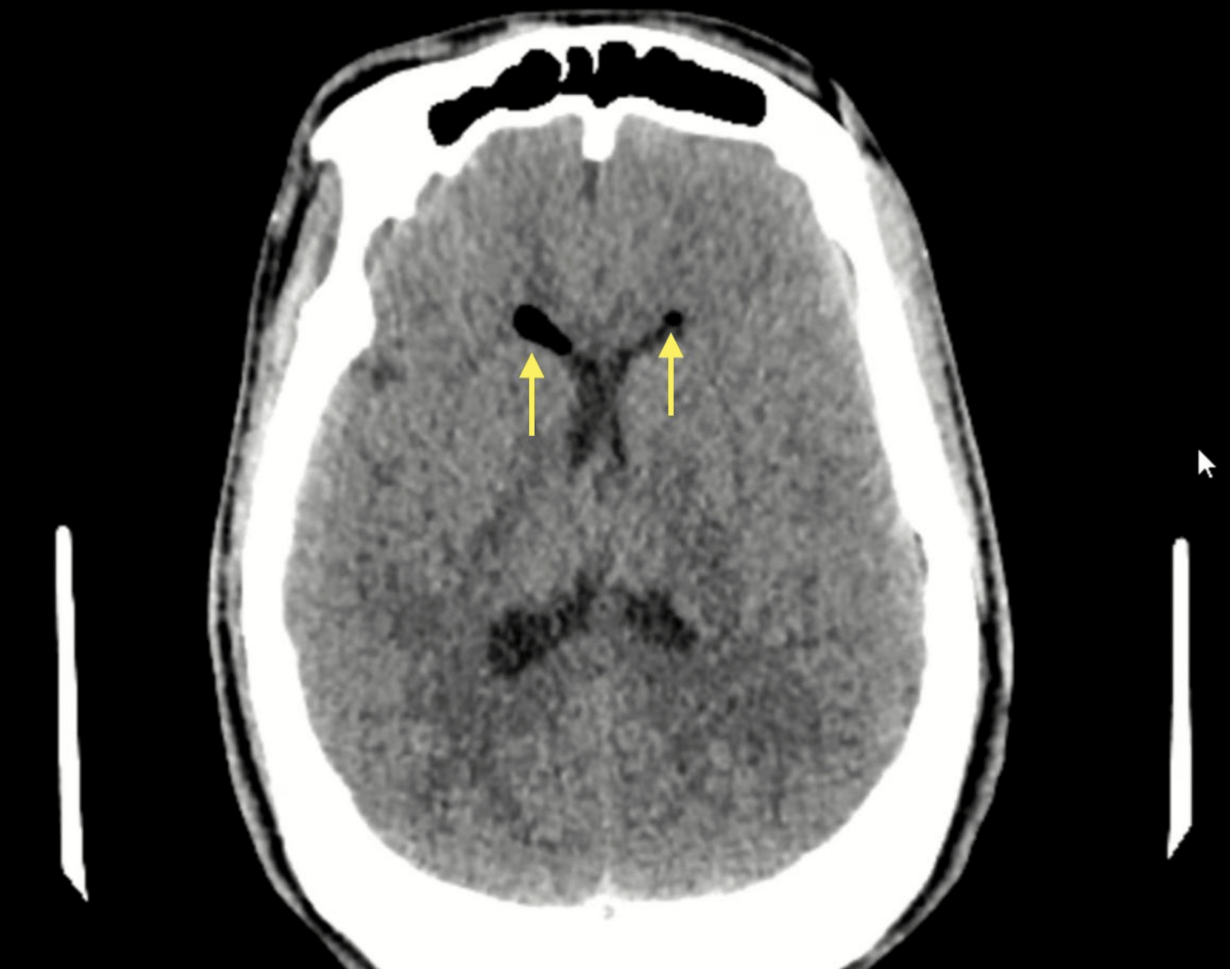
Non-contrast head CT scan Axial CT image showing minimal intraventricular pneumocephalus (yellow arrow) without evidence of hemorrhage or acute parenchymal injury.
